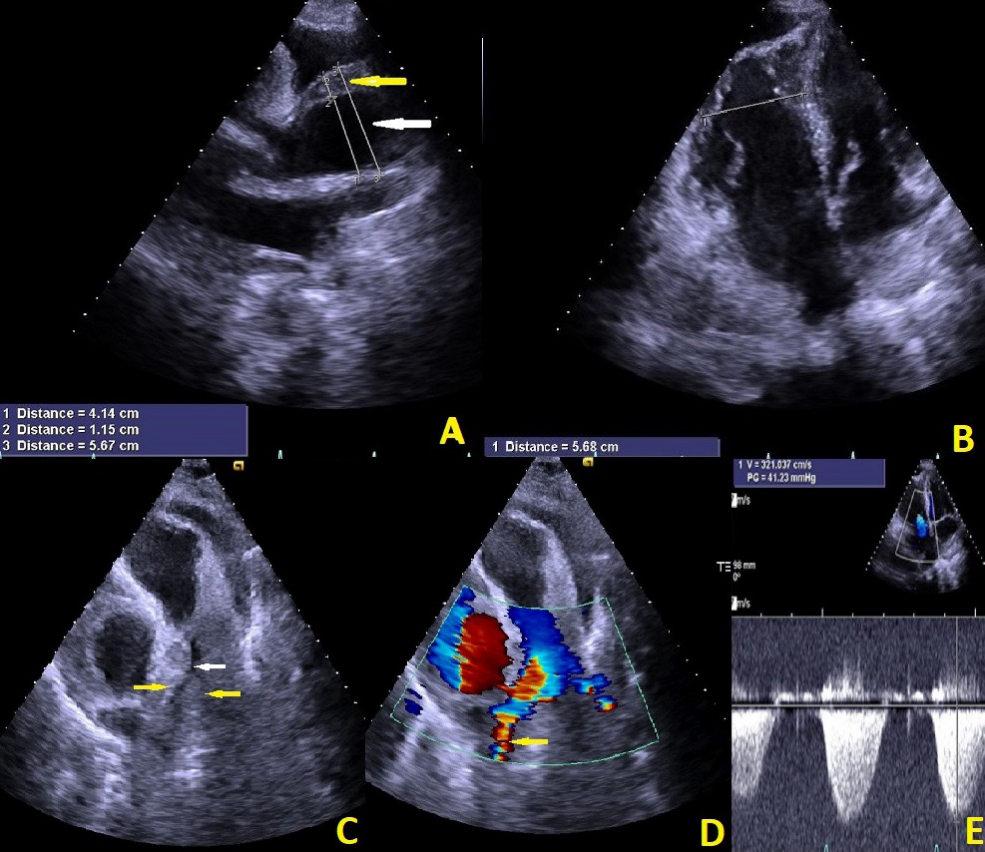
For better evaluation of aorta, thoracic and abdominal aortic computed tomography (CT) angiography was done which supported echocardiographic findings. Ascending aorta was dilated with 70mm diameter. The double lumen aorta with intra-mural contrast, confirmed a type A aortic dissection with 14mm thickness of hematoma. Suspicious evidence of contrast extravasation around dissected aorta was seen. Severe compressions of the distal main and right pulmonary artery by hematoma were seen (Figure 3). Emergent cardiac surgery consult was done and the patient underwent surgery in another center. The Bental surgery was done and the diagnosis was confirmed through the surgery. Due to severe right ventricle systolic dysfunction, the patient’s extubation was very difficult. The patient died ten days after the operation due to multiorgan failure.
